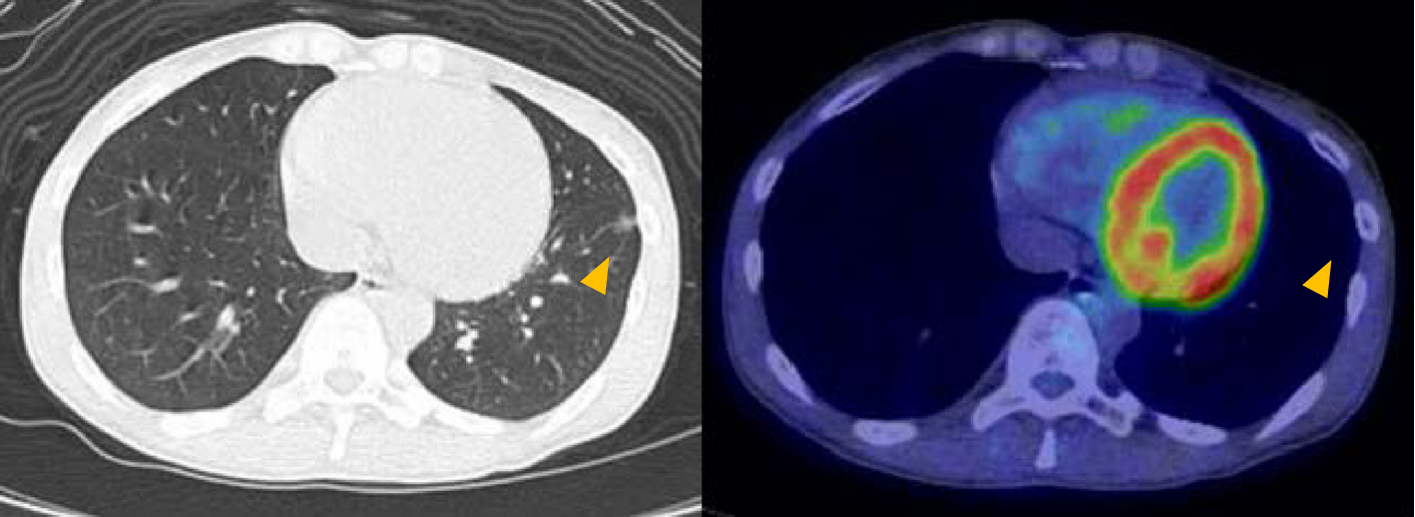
Positron emission tomography-CT showed no abnormal accumulation in the nodule in the left lower lung. Yellow allowheads indicate the location of tumor
